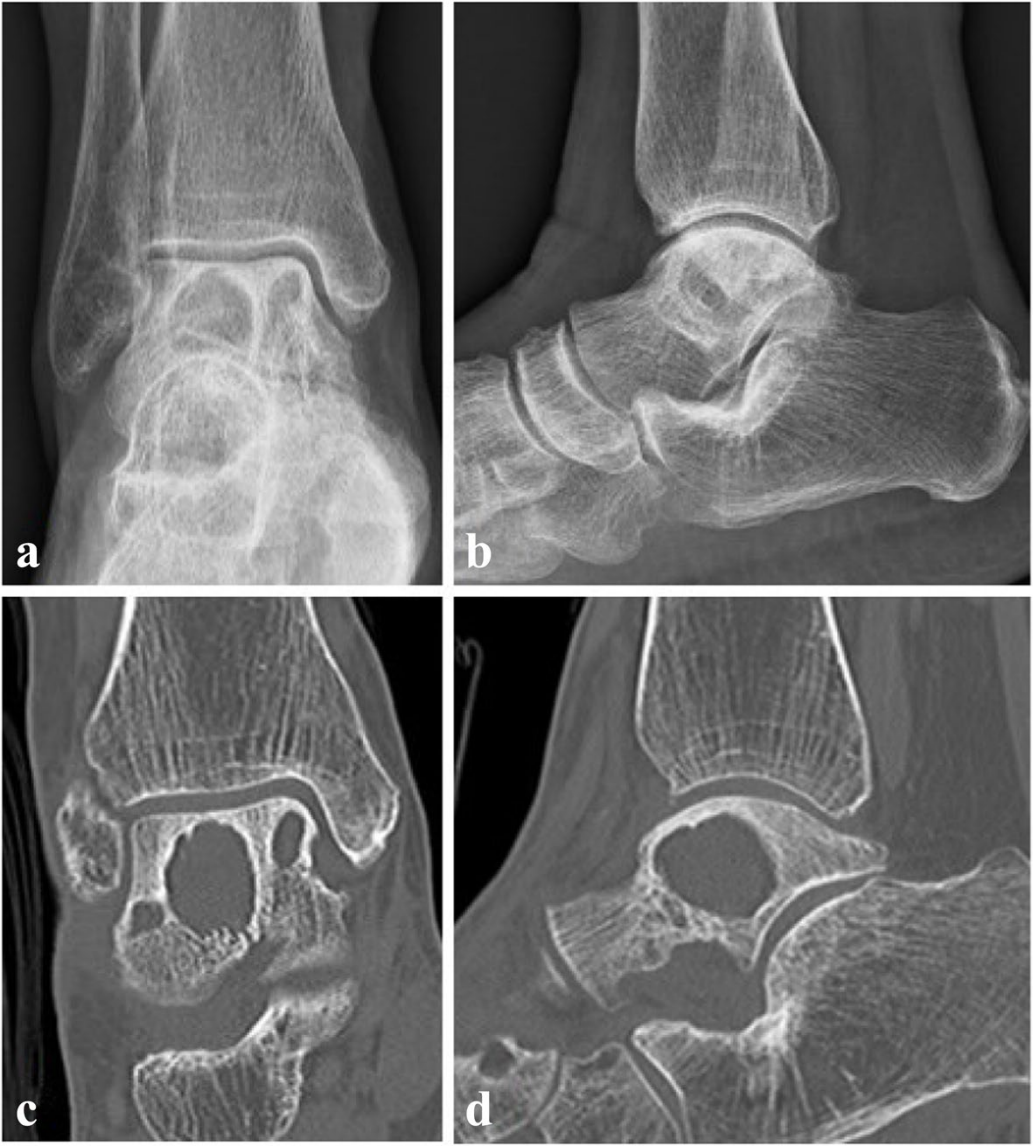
Plain radiographs and CT images of postoperative 10 months. Plain radiographs (a, b) and CT (c, d) showing stationary cystic lesions of talus without definitive progression
